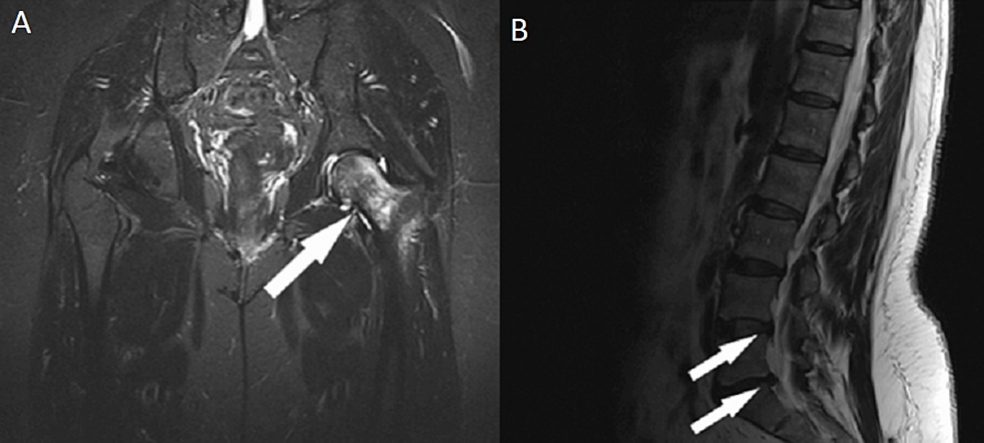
MRI: (A) Coronal plane, findings of avascular osteonecrosis of the left femoral head, and (B) sagittal plane, asymptomatic disc herniations at L4-L5 and L5-S1
